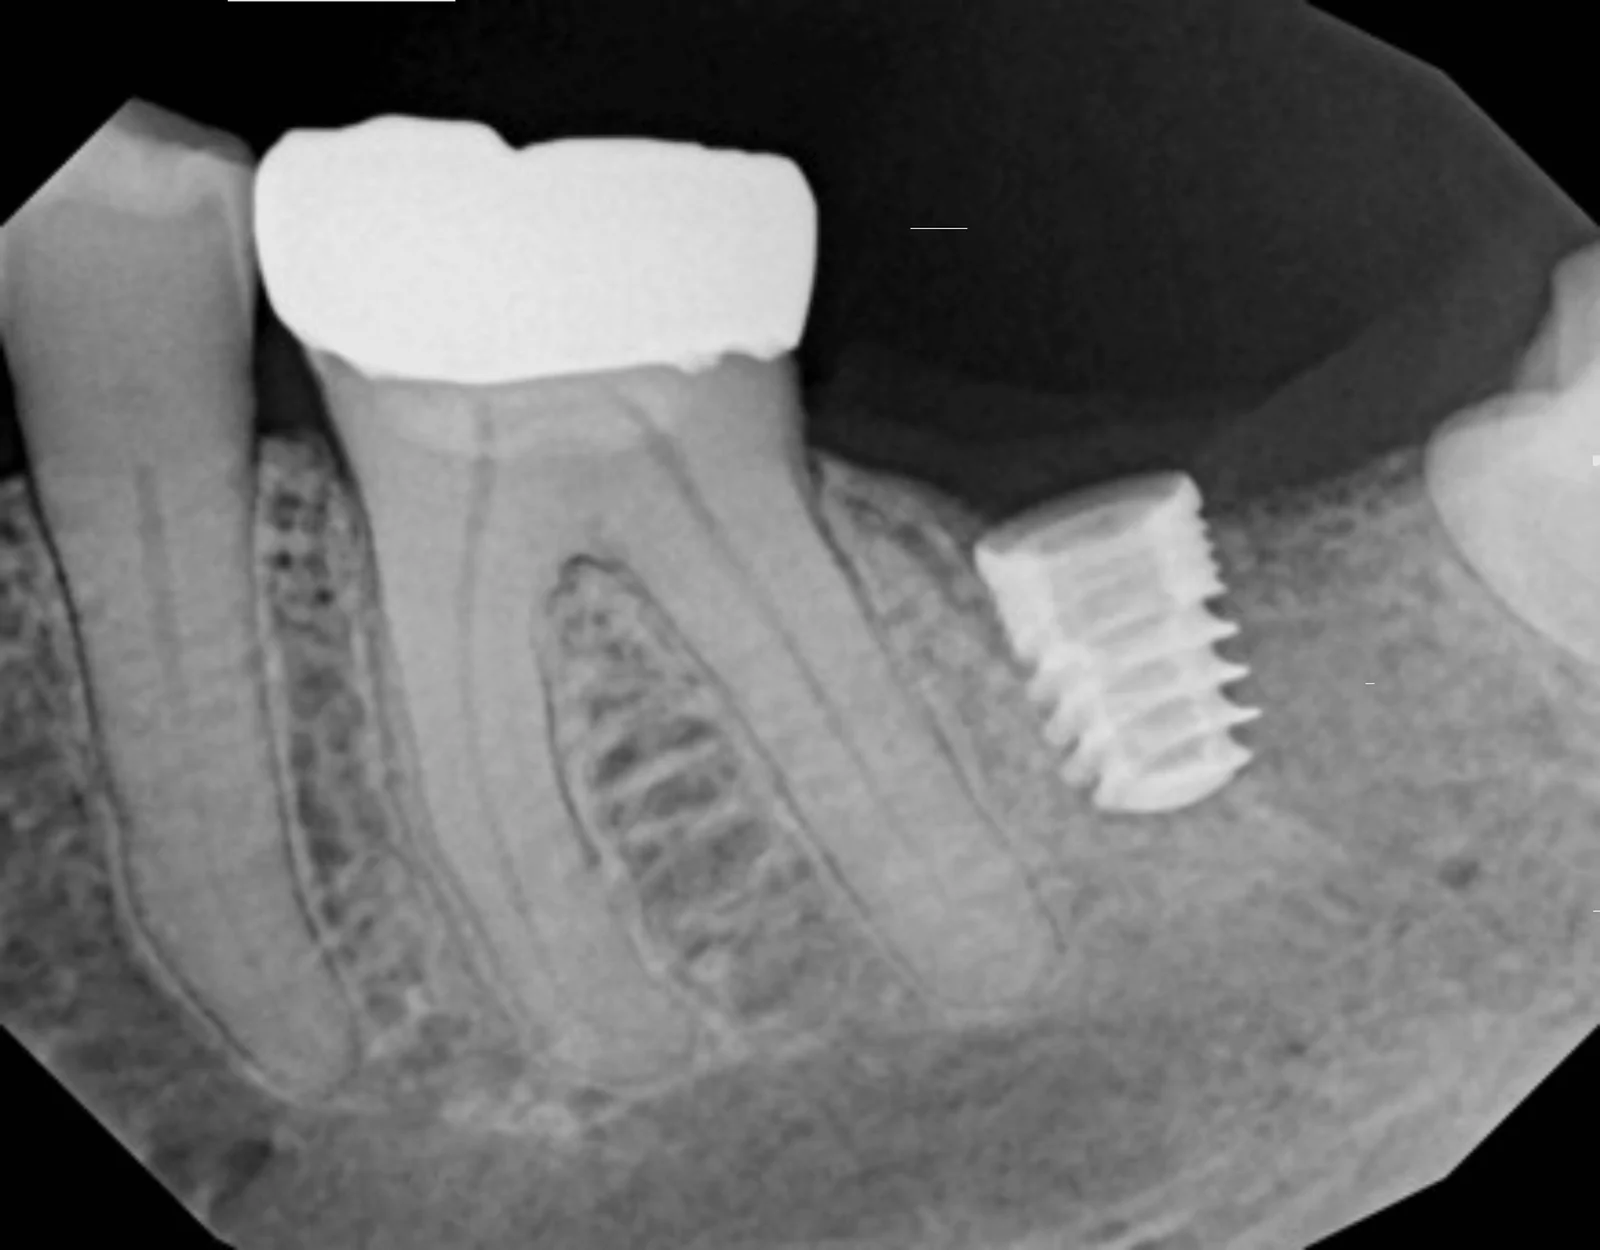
Conventional periapical radiograph following placement of a mandibular implant. While the radiograph demonstrates the implant position relative to the adjacent tooth and crestal bone, it cannot accurately evaluate the buccolingual angulation of the implant or its relationship to the inferior alveolar canal.
